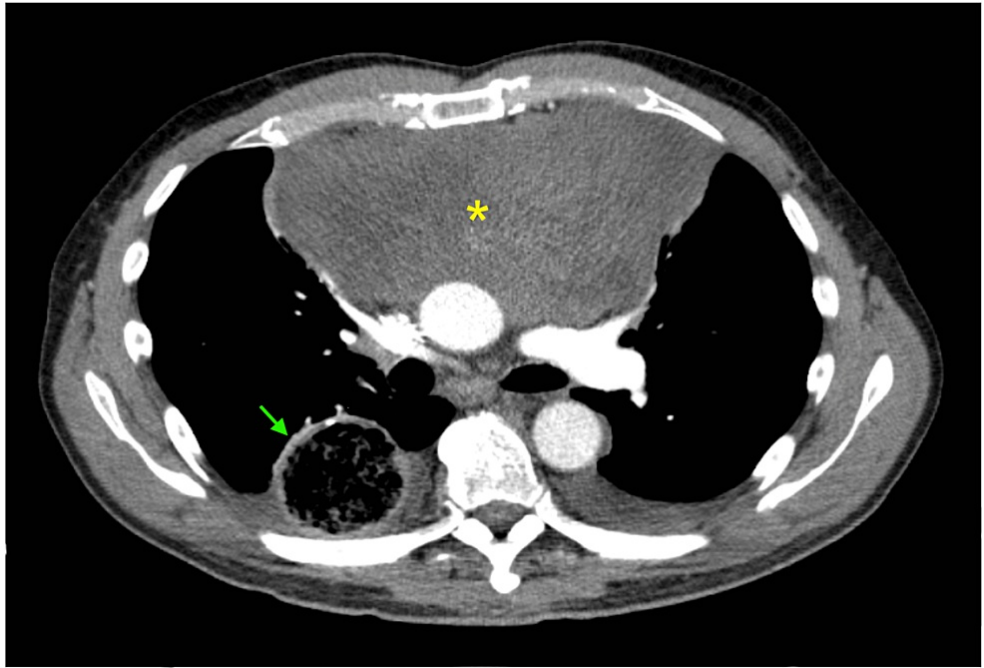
Chest CT, transverse view The yellow asterisk (*) indicates an anterior mediastinal mass. The green arrow indicates gastric pull-through remnants.
